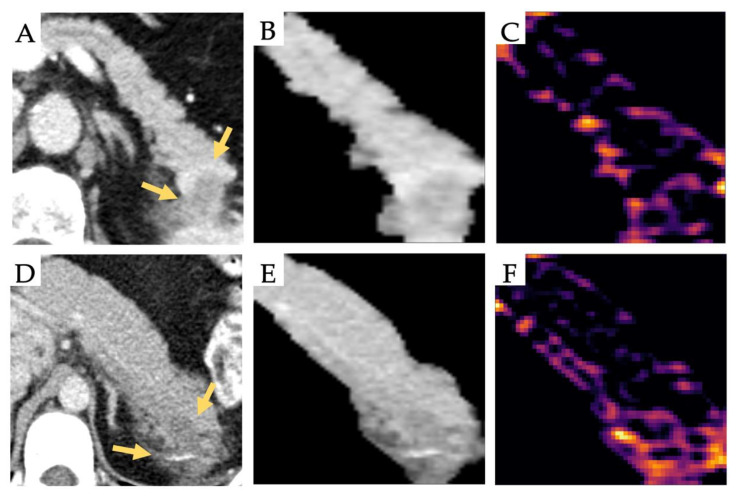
Representative CT-images (Yellow arrows pointing to the lesion) of a pancreatic ductal adenocarcinoma (PDAC) (A) and an autoimmune pancreatitis (AIP) (D) and their corresponding segmentation image (B,E) and feature map (C,F). For the AIP lesion, the activation of the feature map appears to focus on the internal structure; in contrast, activation is noted at the transition zone between the lesion and normal tissue for the PDAC.
